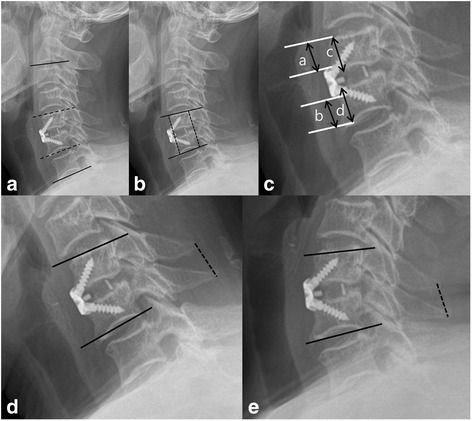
a Cervical alignment was formed by the lines along the lower endplate of C2 to the lower endplate of C7 in the neutral position. The segmental angle was assessed using the Cobb angle of the vertebral bodies adjacent to the involved disc. b The disk height was the distance from the lower endplate of the cephalad vertebral body to the upper endplate of the caudal vertebral body of the fusion segment was measured. And disc height was measured as the average value of the height of the anterior portion and posterior. c The cephalad and caudal plate-to-disc distance (PDD) and the PDD/anterior body height (ABH) ratio were measured. d The segmental angle was measured using the Cobb angle on flexion X-ray. The interspinous distance was measured on flexion X-ray across the fusion segment. e The segmental angle was measured using the Cobb angle on extension X-ray. The interspinous distance was measured on extension X-ray across the fusion segment
